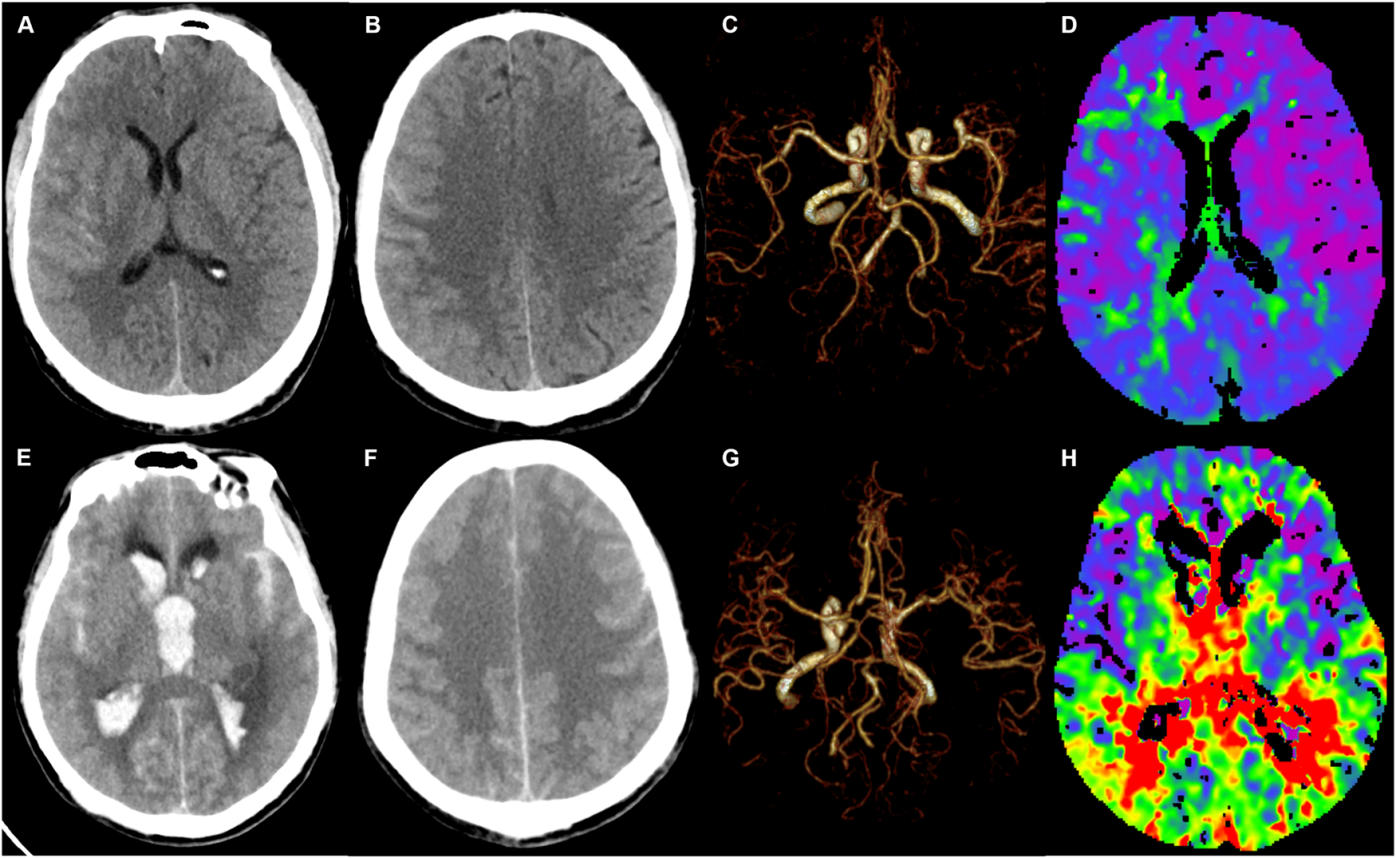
One-stop whole-brain CT perfusion images of patients with and without delayed cerebral ischemia (DCI). A 53-year-old male with evidence of DCI. A, B Non-contrast computed tomography (NCCT) demonstrated a modified Fisher Score (mFS) of 1 and a Subarachnoid Hemorrhage Early Brain Edema Score (SEBES) of 1, with no signs of intraventricular hemorrhage (IVH), intracranial hematoma, or acute hydrocephalus. C Computed tomography angiography (CTA) confirmed the presence of a right middle cerebral artery aneurysm. D TMax perfusion imaging revealed comparatively lower perfusion in the right cerebral hemisphere relative to the left. A 62-year-old male patient presented with DCI. E, F NCCT revealed an mFS of 4 and an SEBES of 4, along with the presence of IVH and acute hydrocephalus. G CTA identified a left posterior communicating artery aneurysm. H TMax perfusion imaging further demonstrated decreased perfusion across both cerebral hemispheres diffusely
